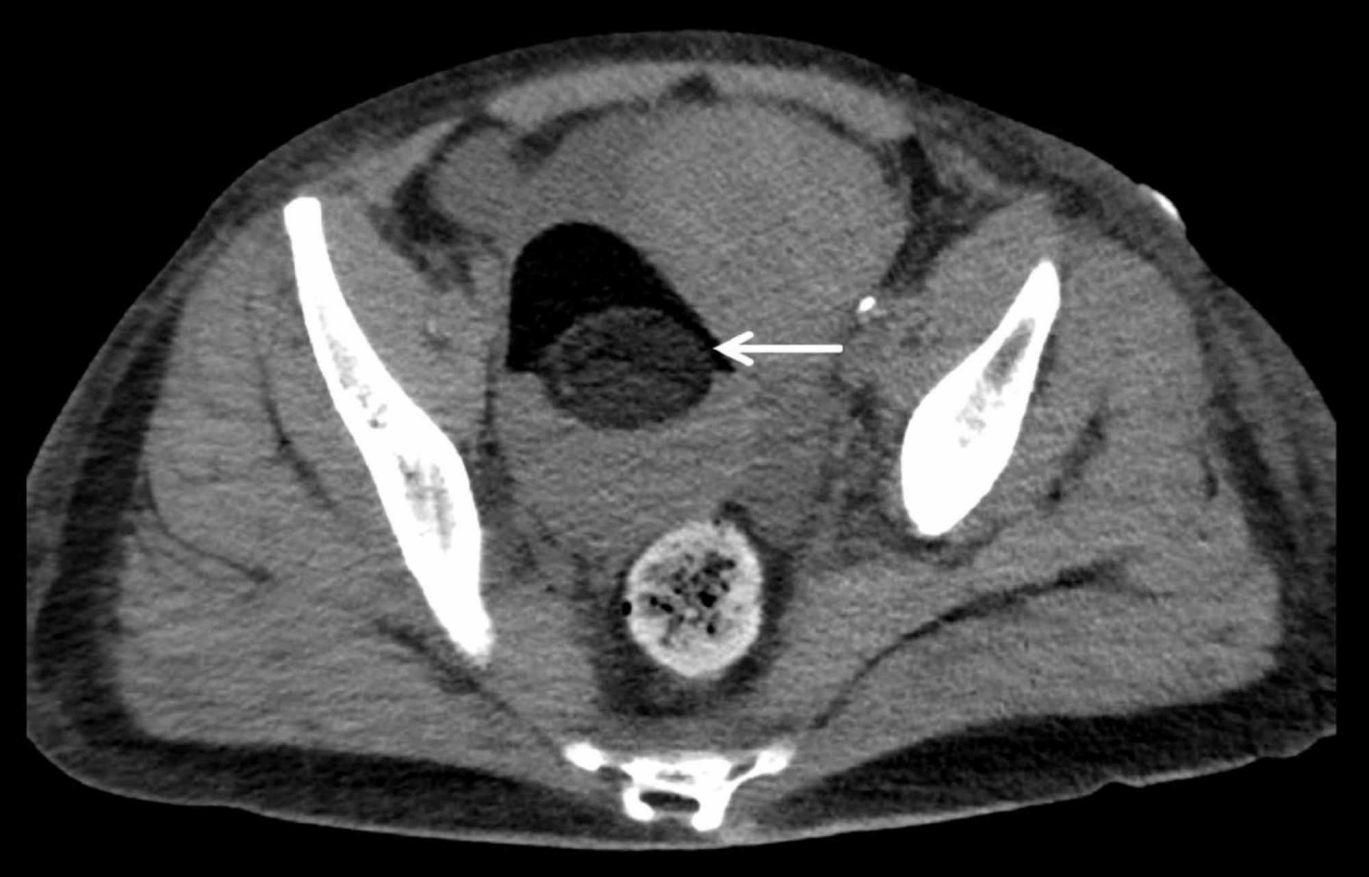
Computed tomography scan of the abdomen and pelvis showing a 9.2 x 6.5 x 7.8-cm mass (arrow) in the right adnexa, likely arising from the right ovary containing fat, fluid, and calcific densities (axial view).
